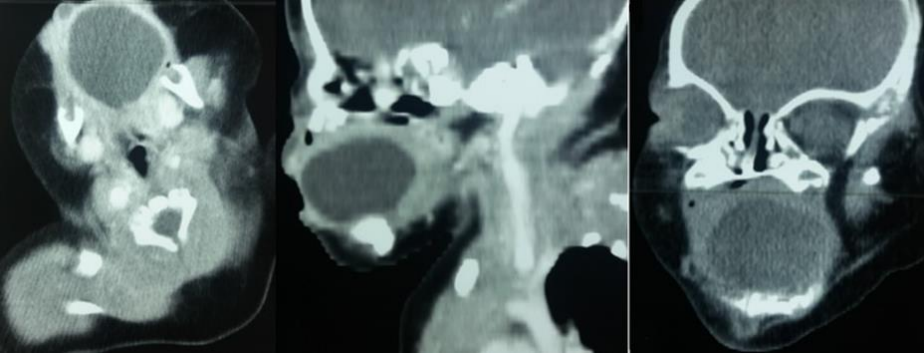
Axial, sagittal and coronal enhanced CT scan showed a large thin-walled cystic mass, which located in the anterior part of the tongue with a homogenous liquid-density content
